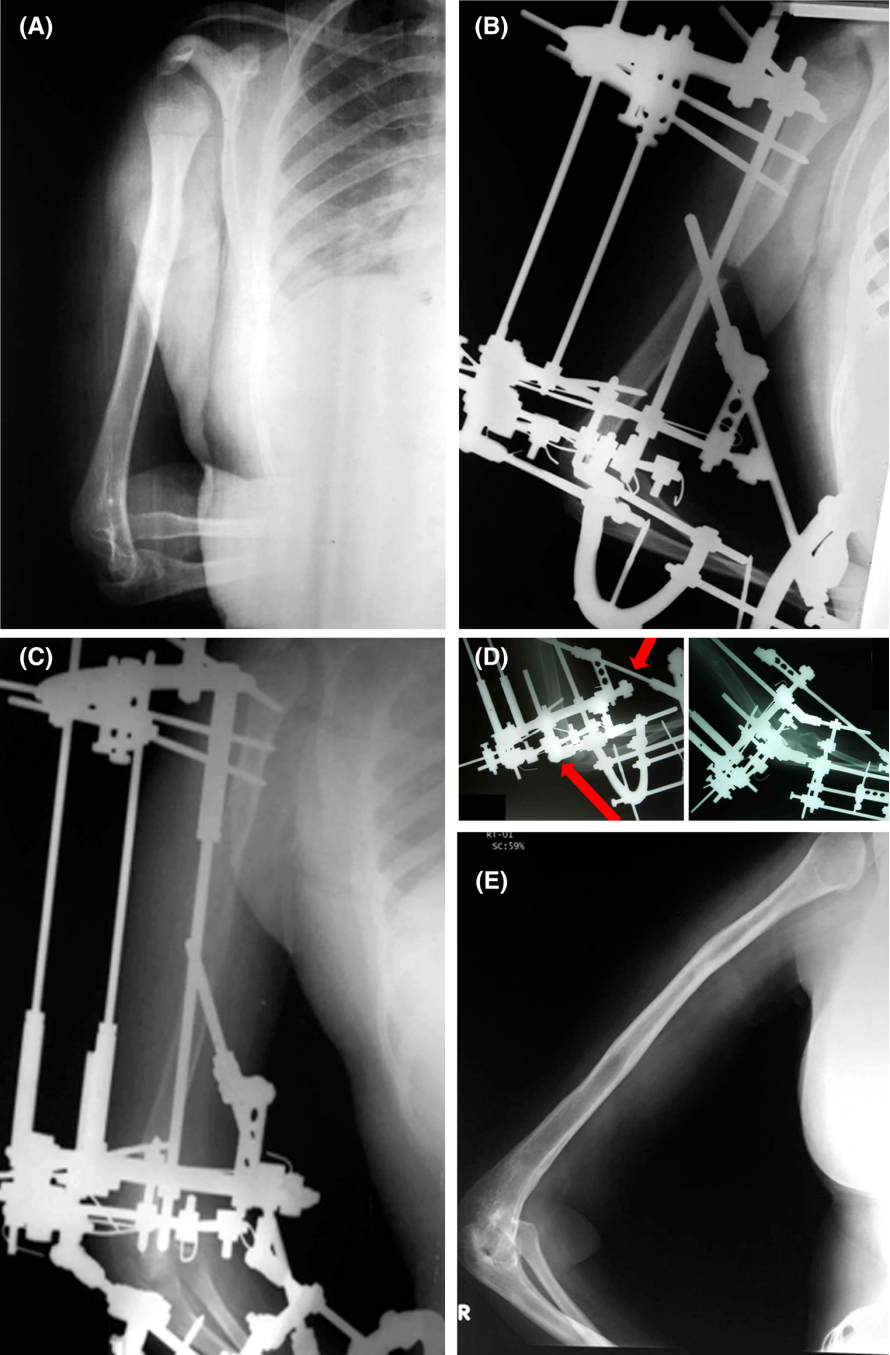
a A 13-year-old girl with Erb’s palsy, 9 cm humeral shortening and fixed elbow flexion (70–100 degrees). b X-ray after frame application to the humeri and forearm. c X-ray at the end of lengthening. d X-ray in maximum flexion and maximum extension. e X-ray at last follow-up 4 years after frame removal
